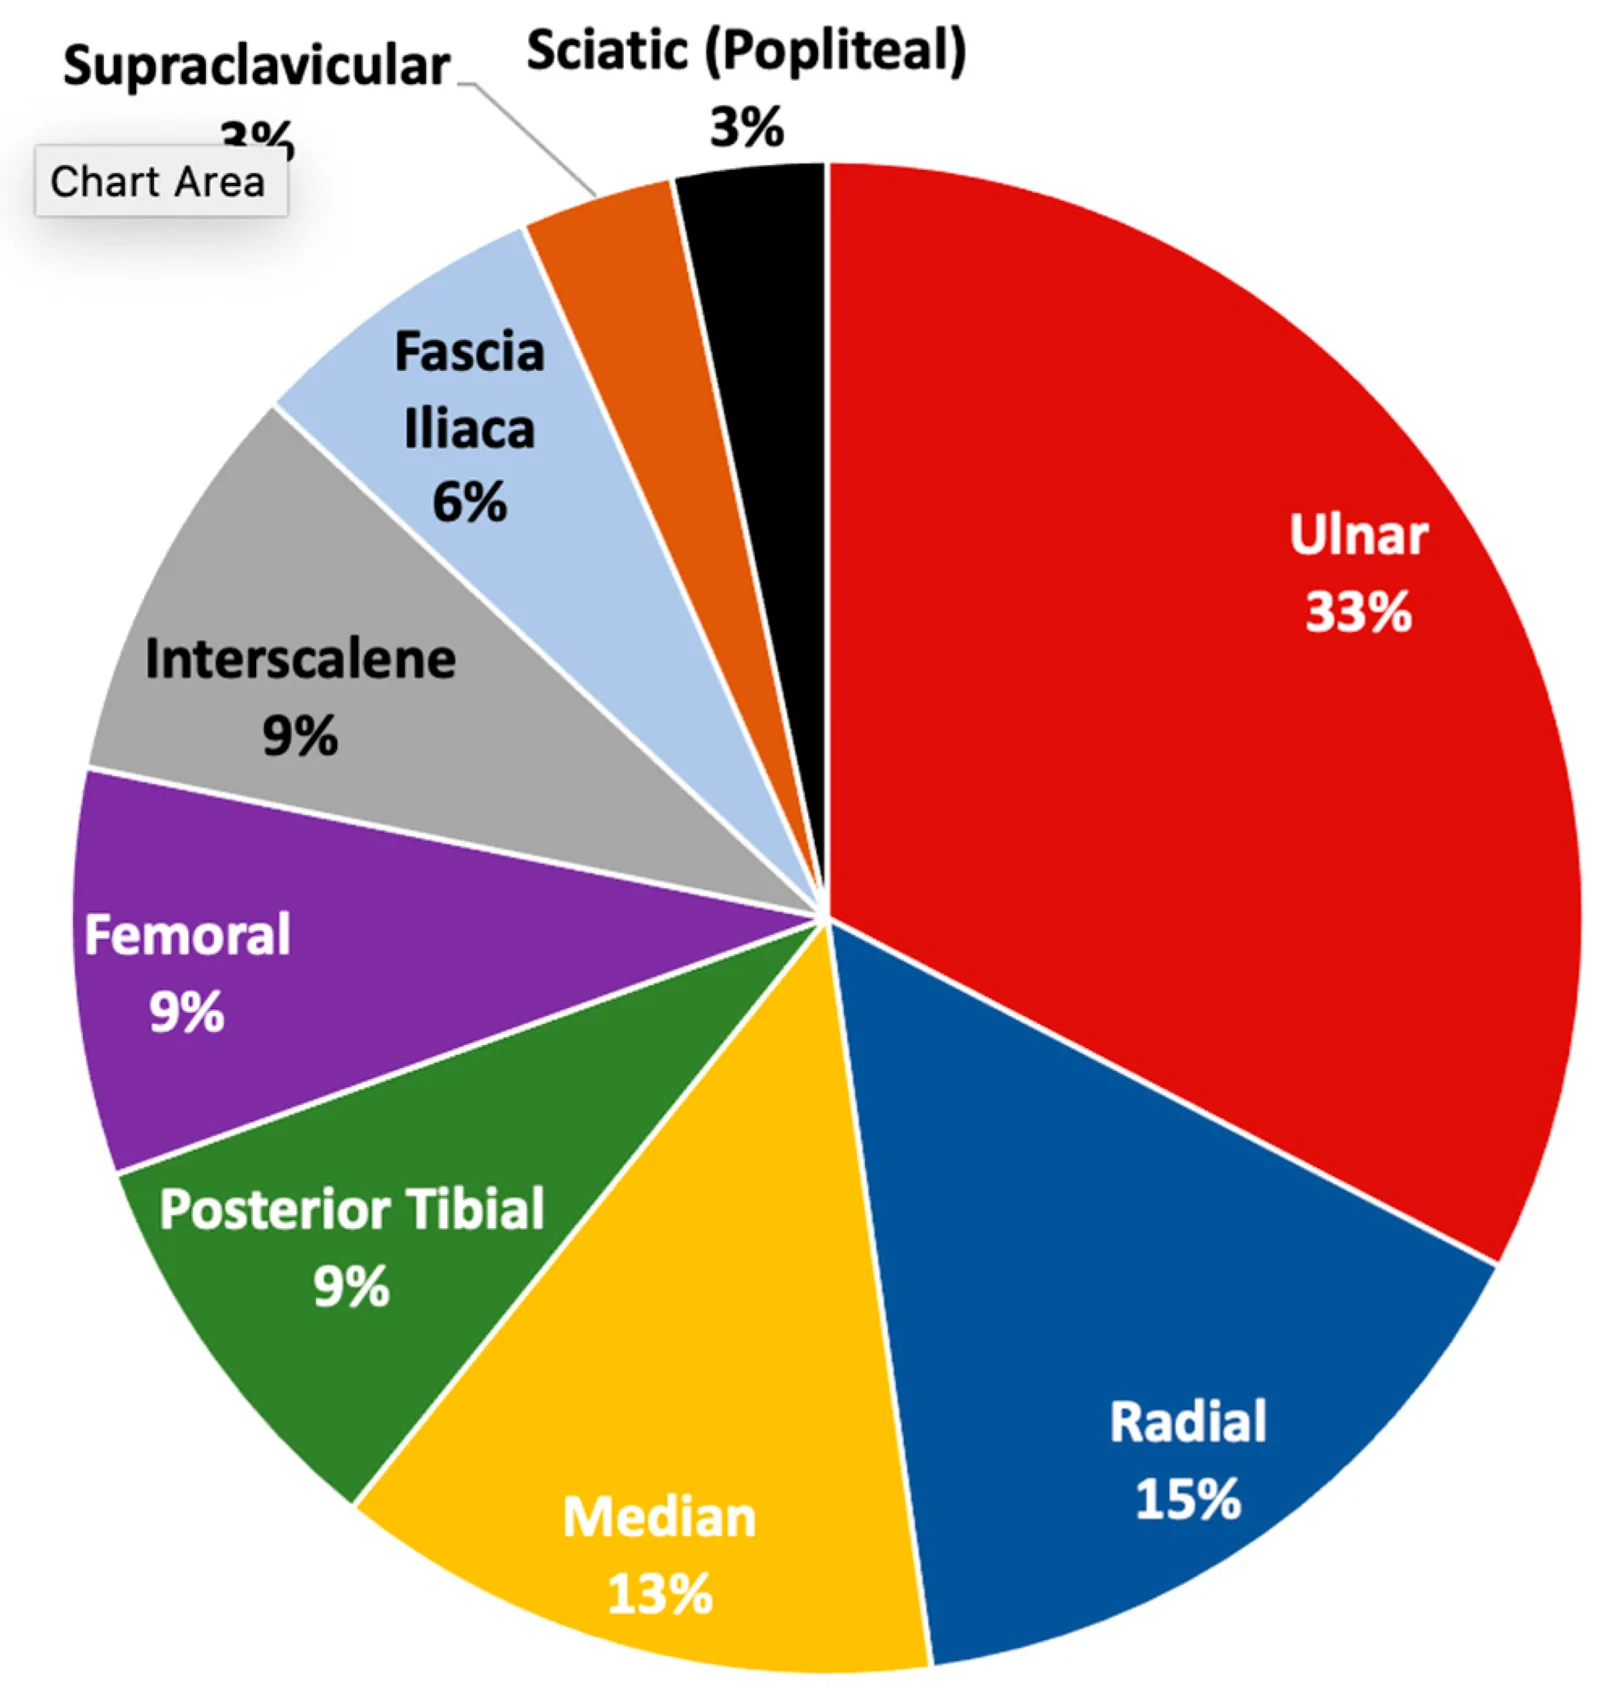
Distribution of ultrasound-guided and ultrasound-assisted peripheral nerve blocks.
